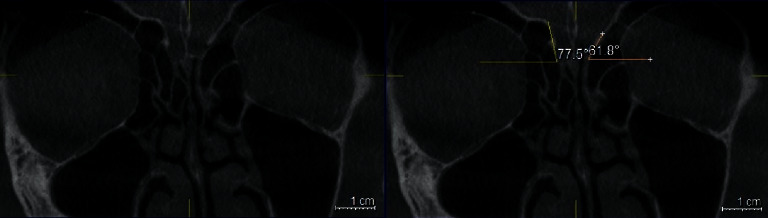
Coronal plan of CBCT demonstrating type II Gera classification on both sides.
